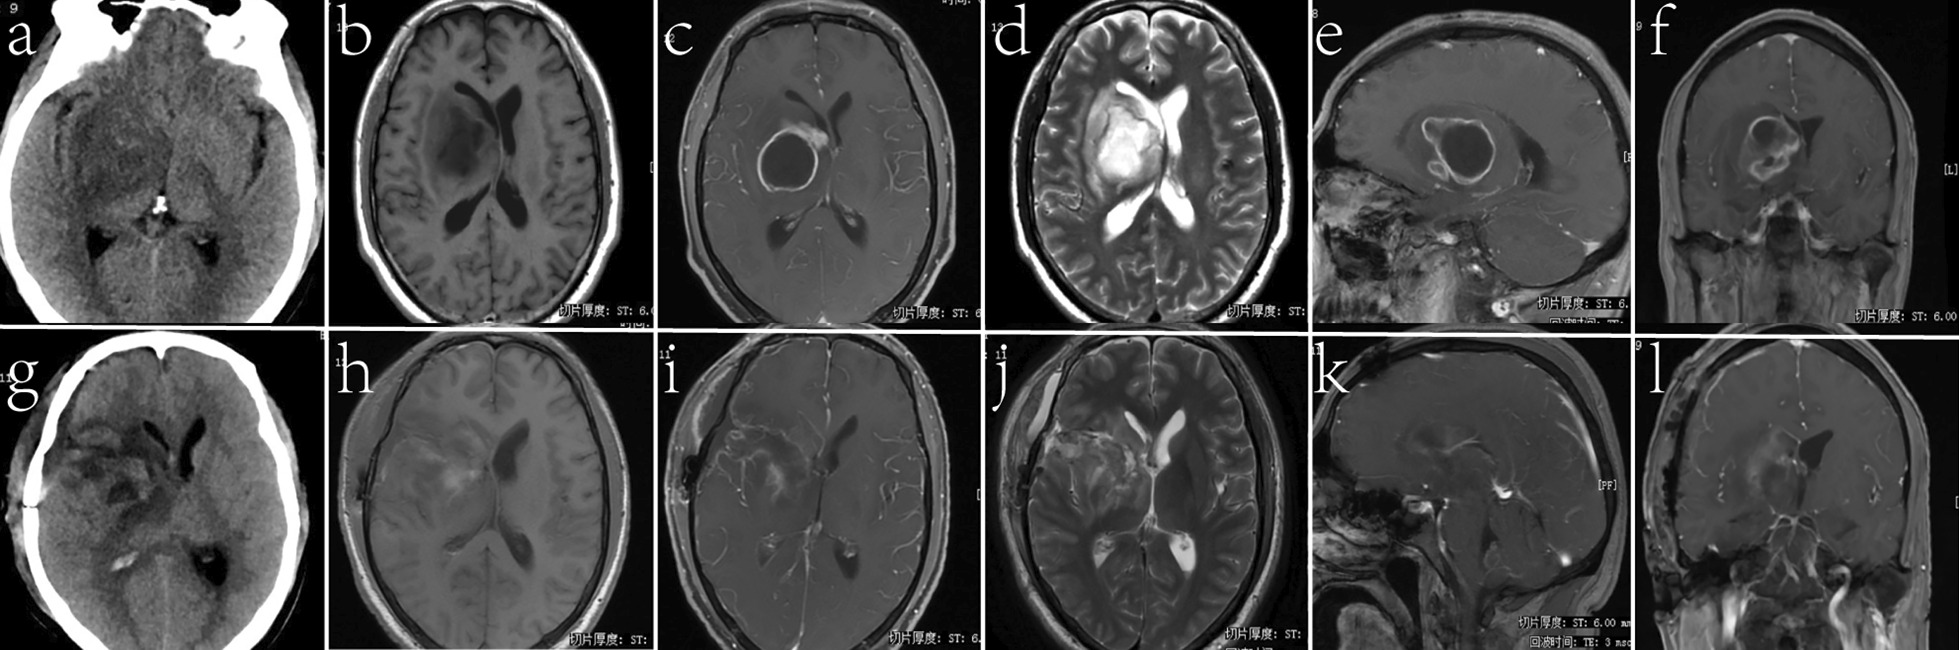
Case 2. Cranial CT and MRI revealed an abscess in the right basal ganglia. Preoperative CT and MRI (a–f). Postoperative CT and MRI confirmed total resection (g–l)
